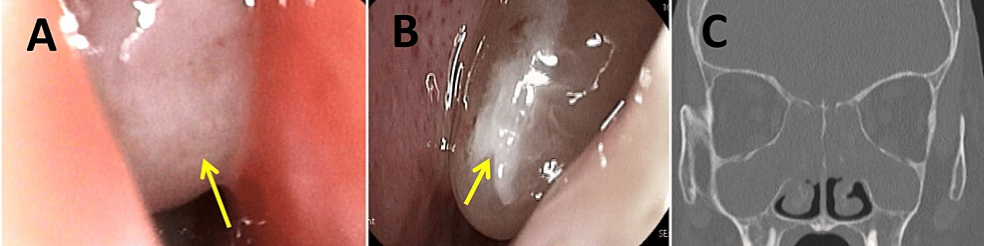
Nasal findings prior to tezepelumab treatment. (A, B) Intranasal findings. Nasal endoscopy at the time of initiation shows nasal polyps on the right side (A) and left side (B), extending from the middle nasal meatus to the inferior margin of the middle nasal concha (yellow arrow). (C) Computed tomography images before tezepelumab treatment. CT scan of the sinuses at initiation revealed soft tissue density lesions in all bilateral sinuses.
